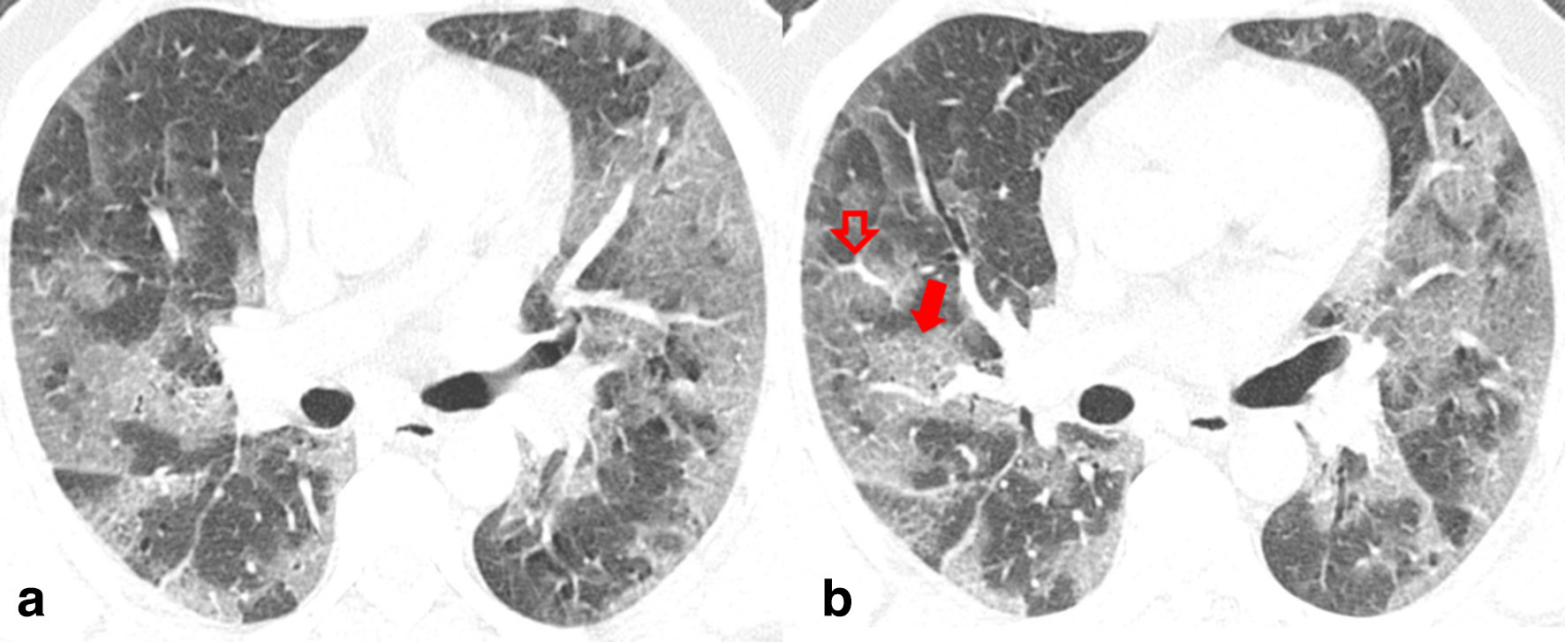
Two consecutive non-contrast axial chest CT images in lung window settings of a 62-year-old male COVID-19 positive patient showing multiple patchy peripherally and centrally distributed ground glass opacities with crazy paving pattern denoted by the solid arrow. Segmental vessel dilatation is also seen, denoted by a hollow arrow. The patient required mechanical ventilation (clinically unstable) and subsequently expired. COVID-19, Corona virus disease 2019
